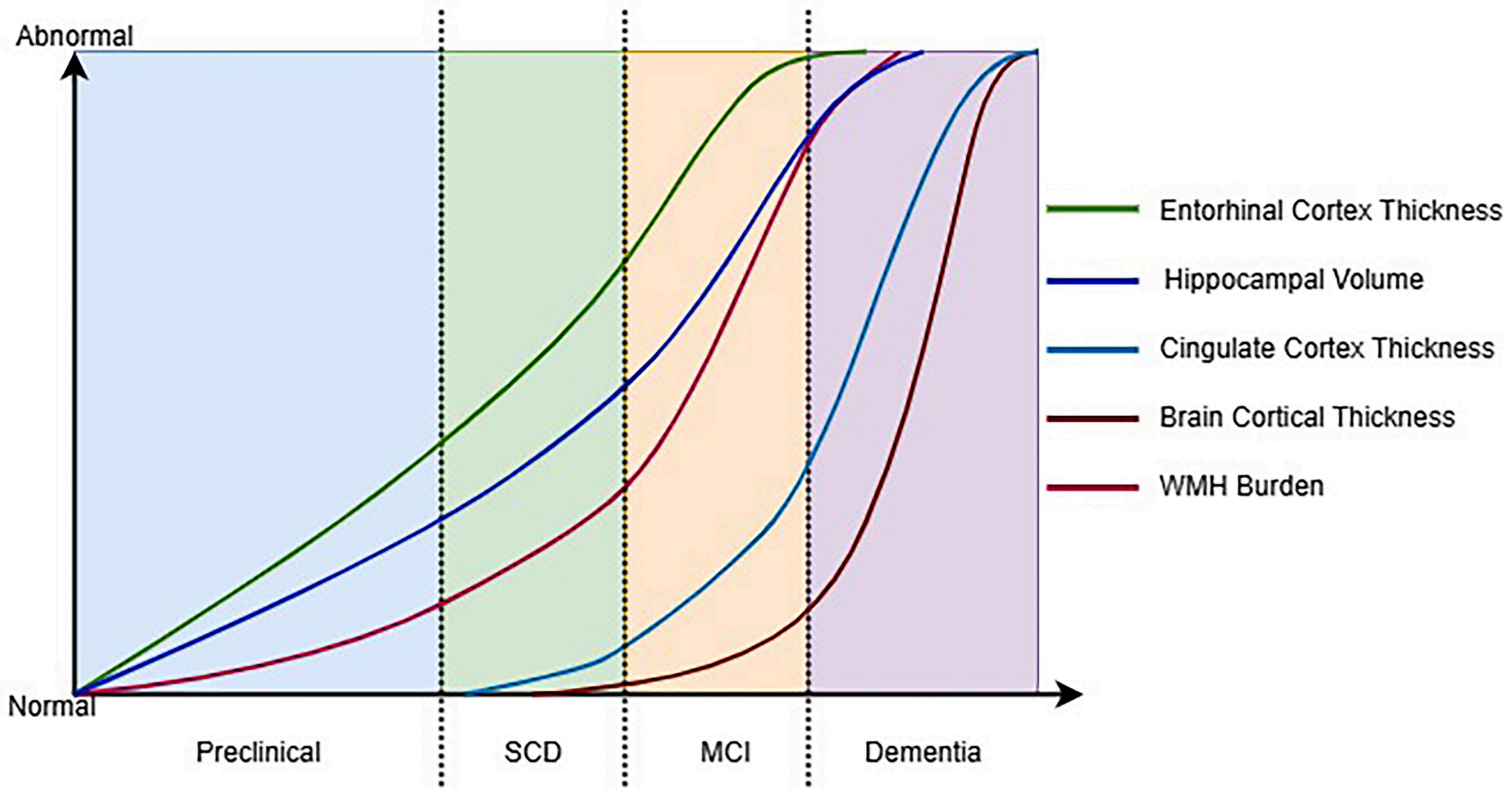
Hypothetical timeline of key structural MRI changes across the AD continuum. SCD, subjective cognitive decline; MCI, mild cognitive impairment; WMH, white matte hyperintensities.
